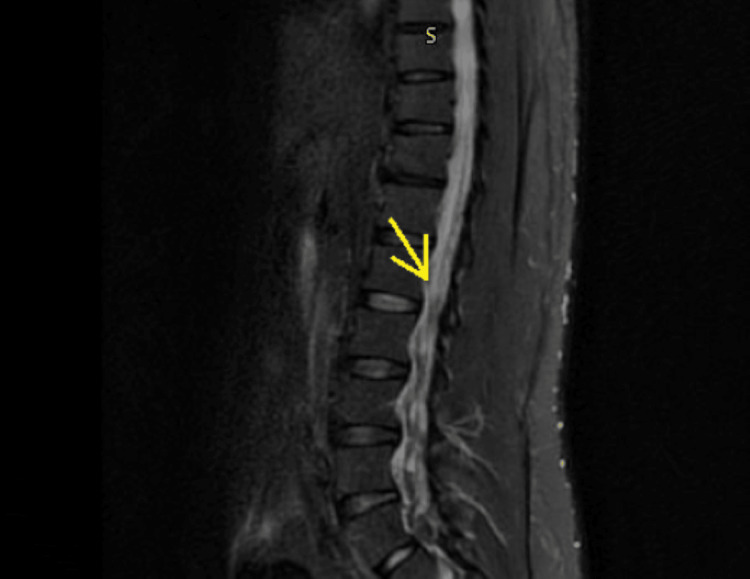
Lumbar spine MRI demonstrating normal alignment without evidence of neural compression, bone fracture, or cerebrospinal fluid (CSF) leak (yellow arrow) S in the picture represents sagittal plane.
